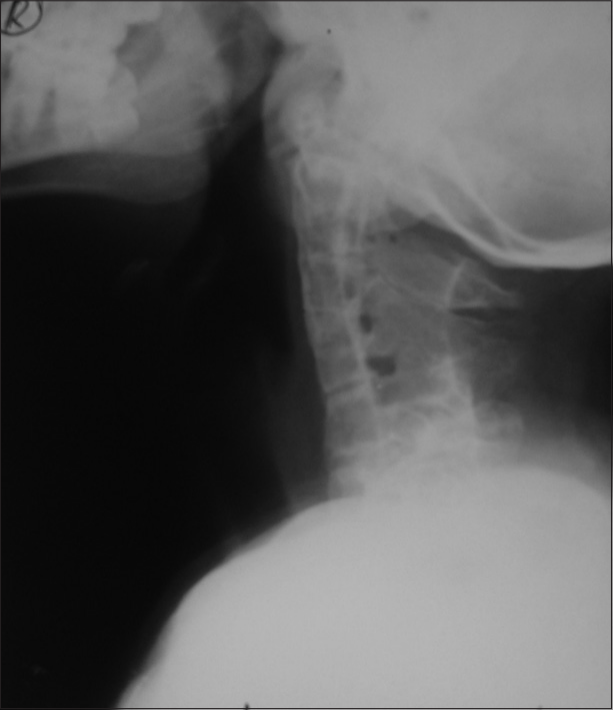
X-ray lateral view of cervical spine showing fused cervical vertebrae
